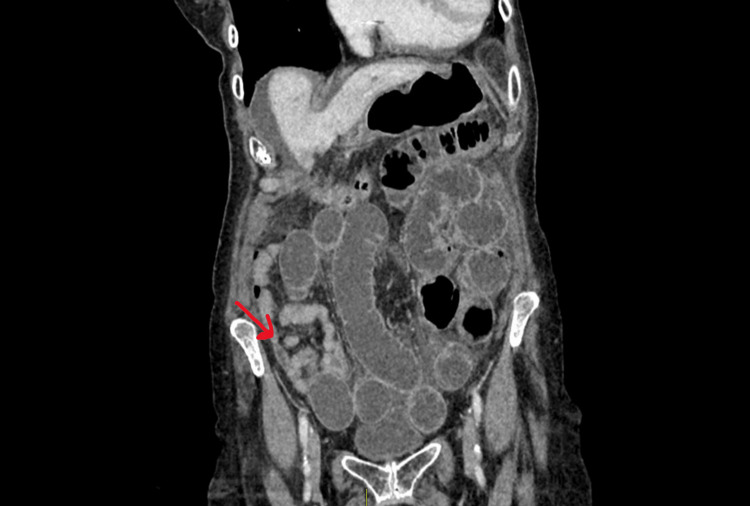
CT showing proximal bowel dilation with distal small bowel collapse with the transition point in the right iliac fossa
